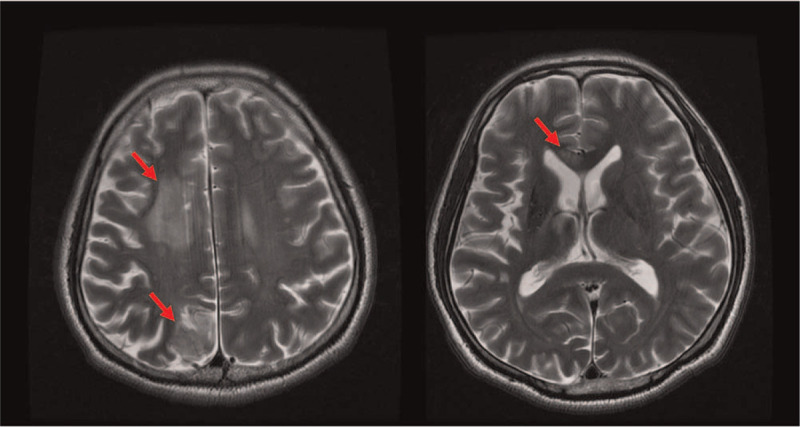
T2-weighted axial brain magnetic resonance images show subacute to chronic infarction (red arrow) in the right frontal lobe (superior frontal and precentral gyri), right parietal lobe, and right corpus callosum.
